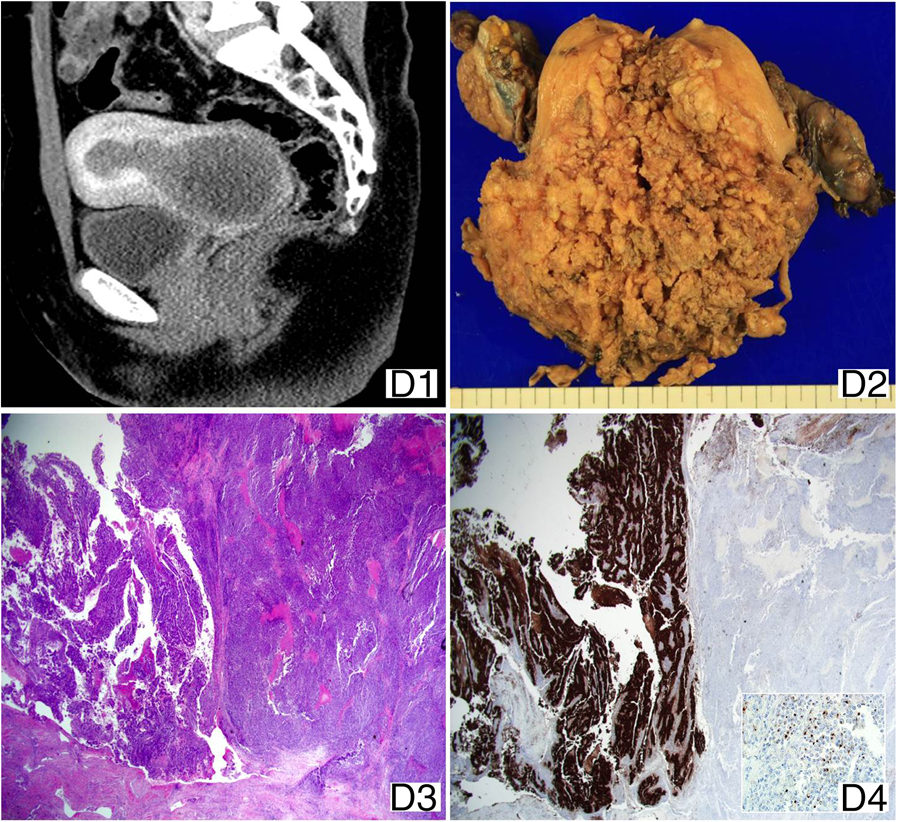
Case 4. (*D1*) Sagittal contrast-enhanced CT shows a bulky hypodense mass filling the endometrial cavity. (*D2*) The large outbulging mass with friable surface. (*D3*, ×2.5) A sharp border between differentiated and UC component and EMA (*D4*, ×2.5 and ×400) shows strong cytoplasmic expression in the former and focal dot and cytoplasmic expression in the latter
